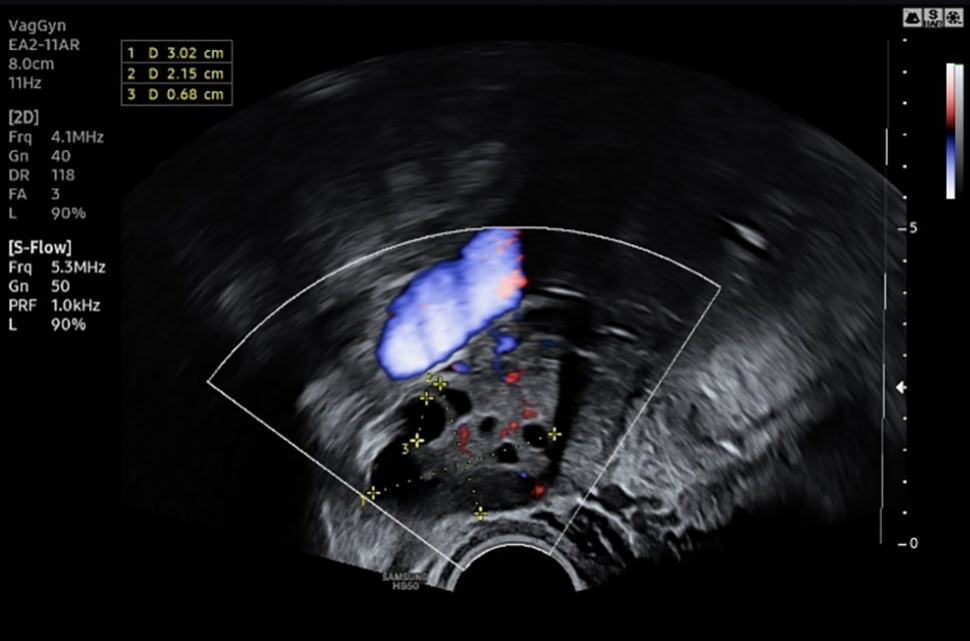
Unremarkable transvaginal sonogram of the right ovary
